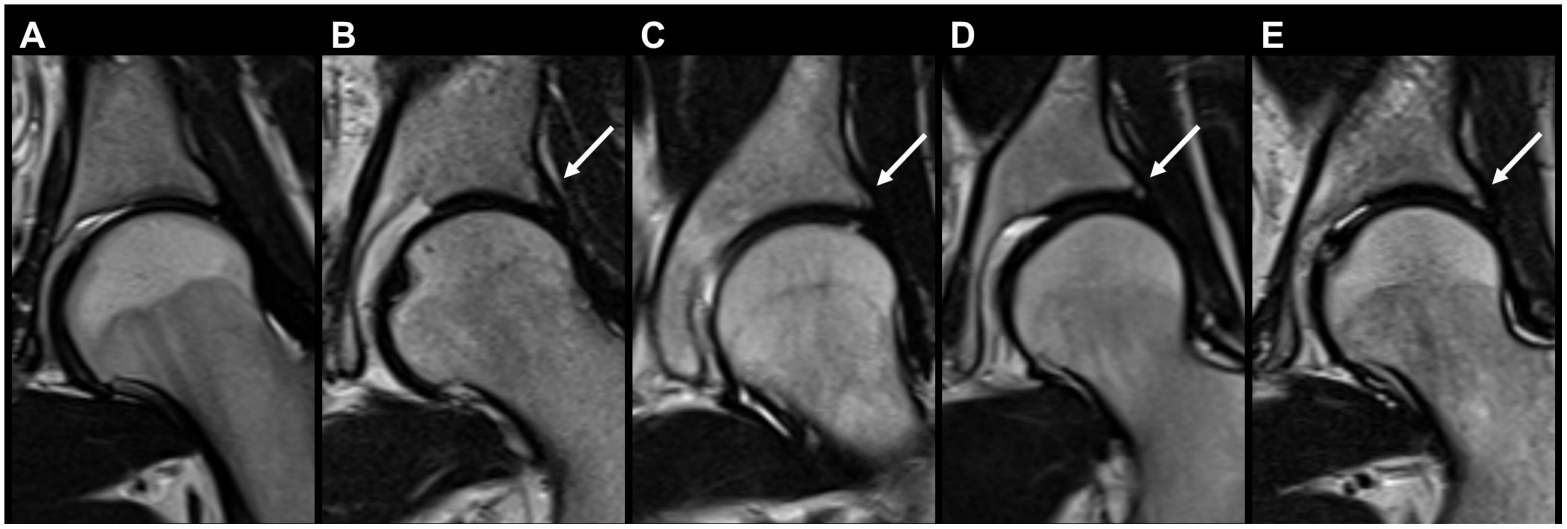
Coronal T2 FSE (fast spin echo) of five different patients showing hip acetabular rim ossifications on MRI for endometriosis (arrows): (A) No ossifications, (B–E) acetabular rim ossification of different sizes in the posterosuperior quadrant.
